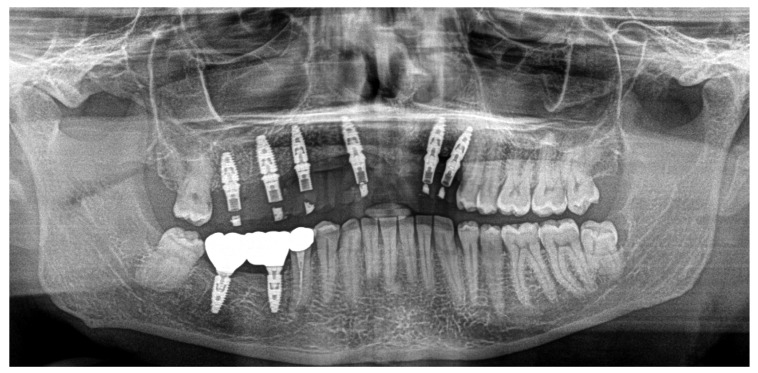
Radiographic follow-up in November 2024.
